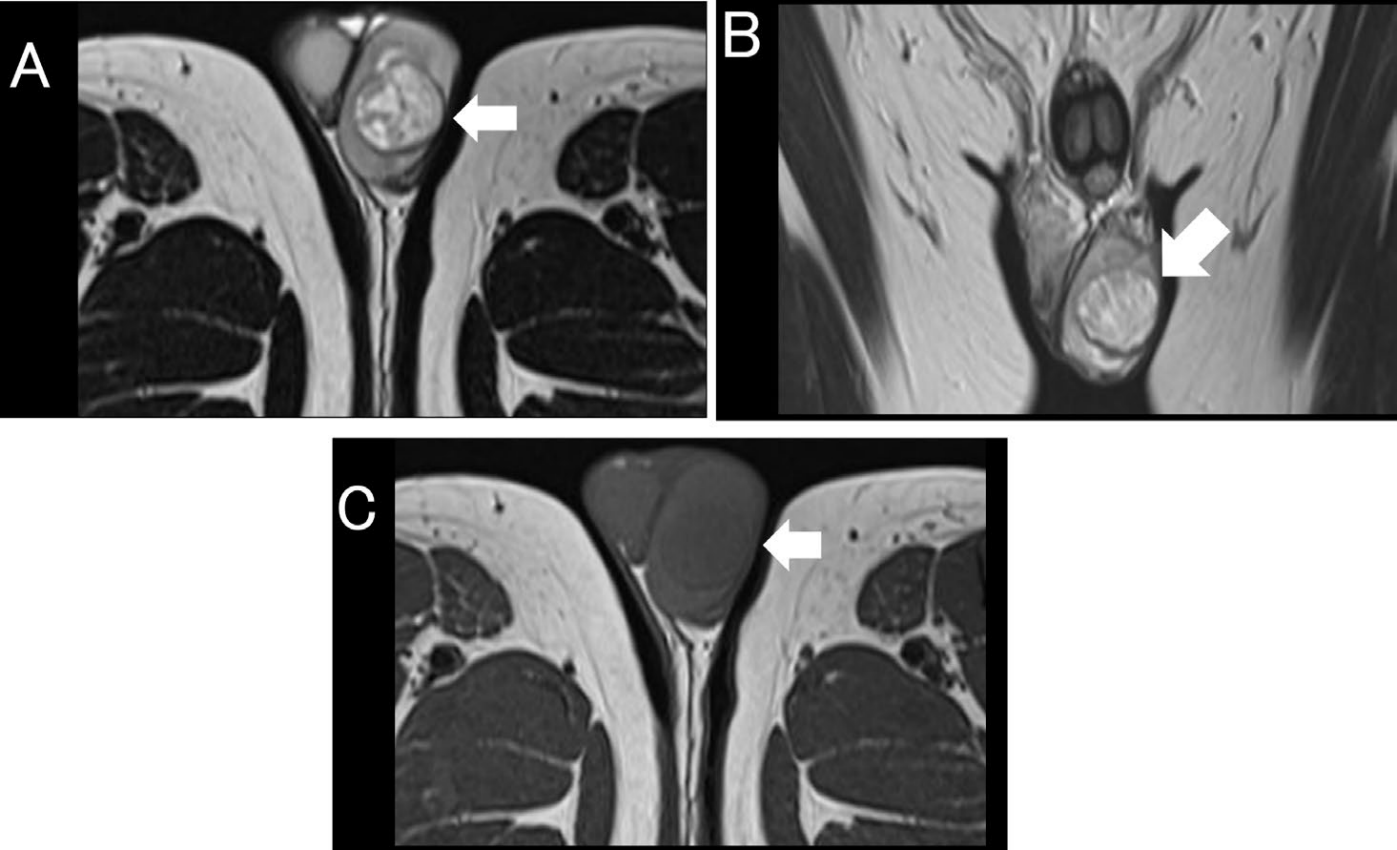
MRI findings of the left testis. (A, B) MRI showed tumor with a heterogenous high‐signal intensity and low‐signal capsule on the axial and coronal T2‐weighted images. (C) The tumor showed a uniform low‐signal intensity on the T1‐weighted MRI axial image.
